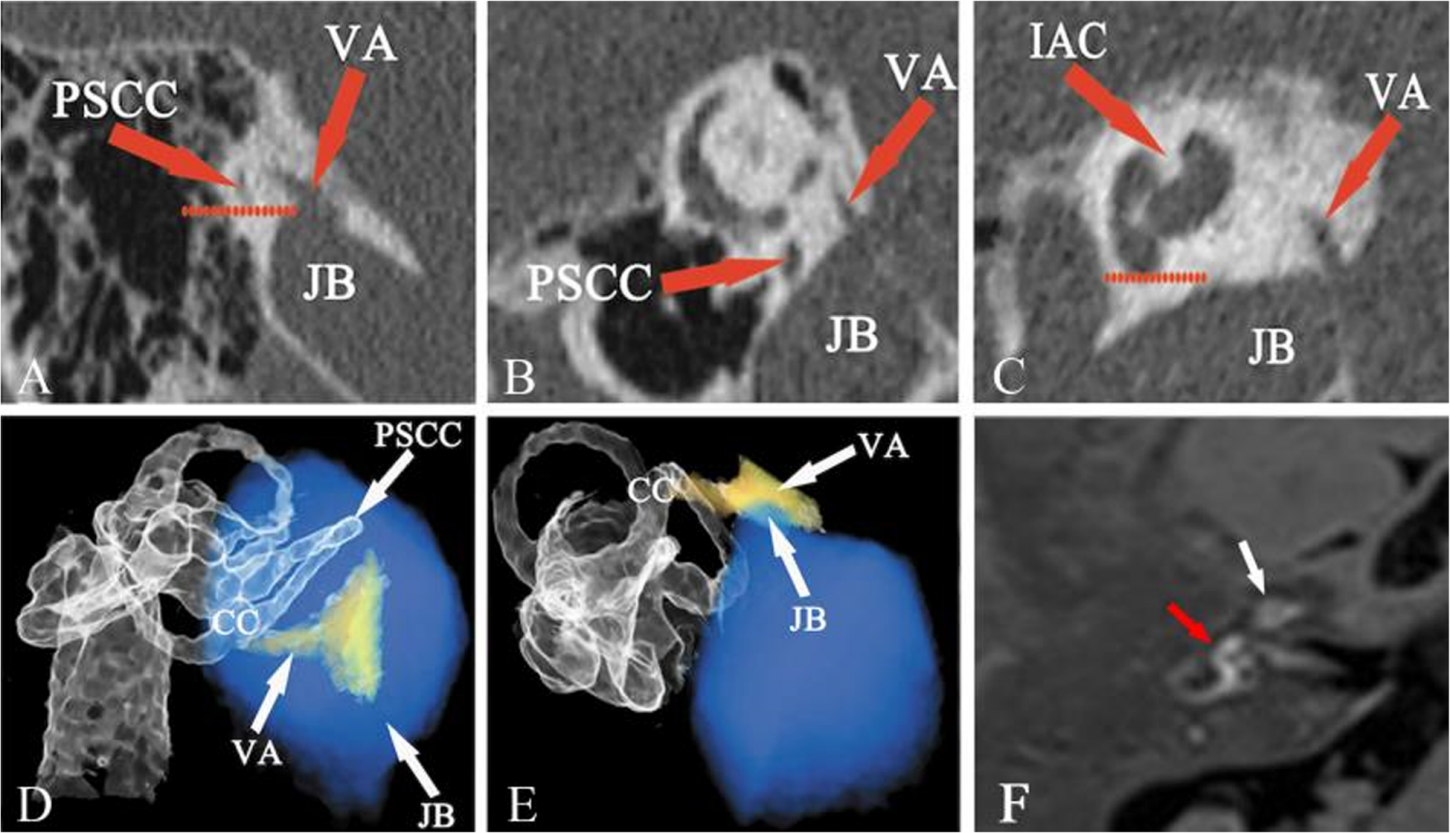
Type 3 JB in a non-hydropic ear. Type 3 is demonstrated in the (a) coronal, b Pöschl and c sagittal planes with the JB above the inferior margin of the PSCC, but below the inferior margin of the IAC, which was imaged at the fundus with the modiolus. A dehiscence was identified between the visualized external aperture of the VA and the upward extension of the JB (a, b and c). Three-dimensional reconstruction computed tomography showing the type II anatomic relationship between JB and VA, where the JB was found to be in close contact with the VA, but the VA was shown intact without obstruction in the (d) posterosuperior and (e) posteroinferior view. f 3D-real IR MRI showing no endolymphatic hydrops in either the cochlea (white arrow) or the vestibule (red arrow), where normal bright perilymphatic fluid was visible. CC, common crus; JB, jugular bulb; JBVAD, JB-vestibular aqueduct dehiscence; PSCC, posterior semicircular canal; IAC, internal auditory canal; VA, vestibular aqueduct; 3D-real IR, three-dimensional real inversion recovery
